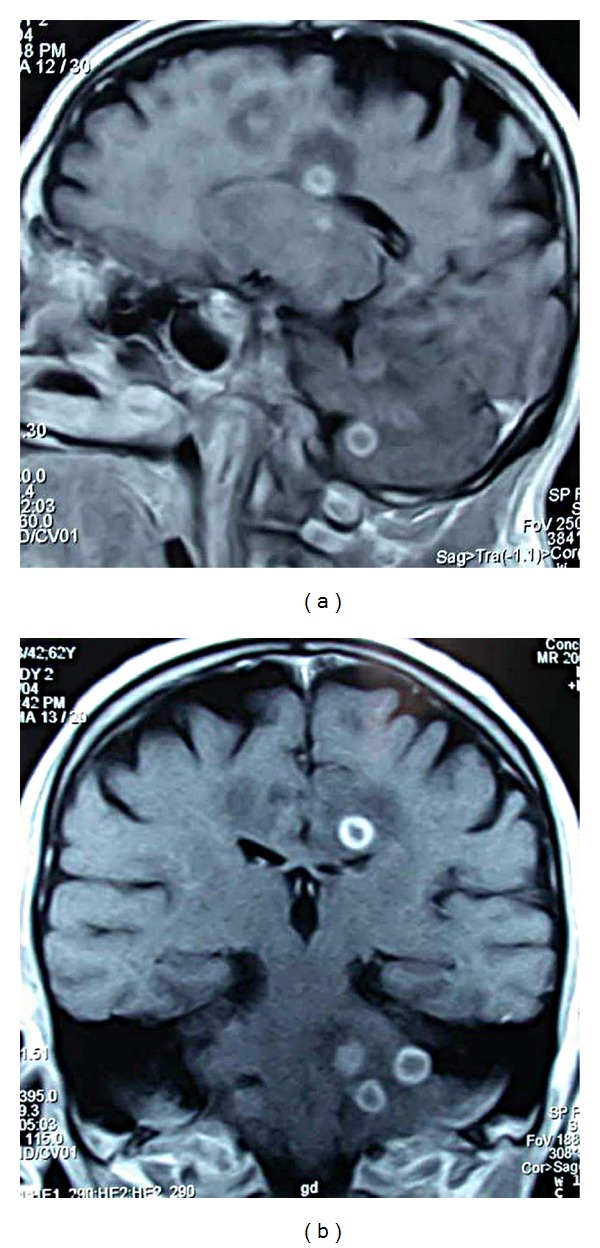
Ring-like contrast-enhancing multiple tuberculomas with a hypointense center and perifocal edema in the posterior fossa, frontal lobe, and around corpus callosum in T1W coronal and sagittal plane as shown with contrast-enhanced cranial MRI.
